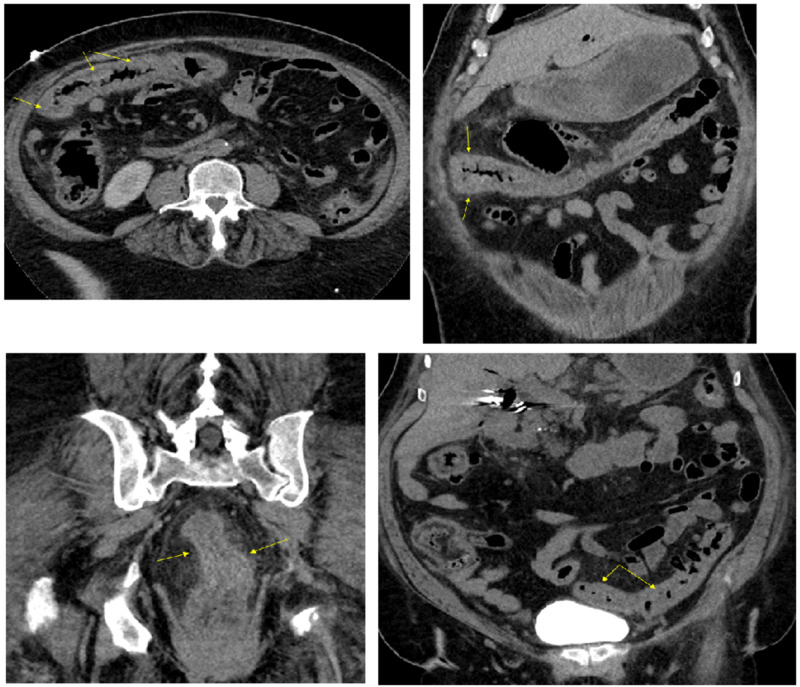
CT of abdomen and pelvis Diffuse mucosal thickening of colon with pericolic stranding suggestive of pancolitis (yellow arrows).
